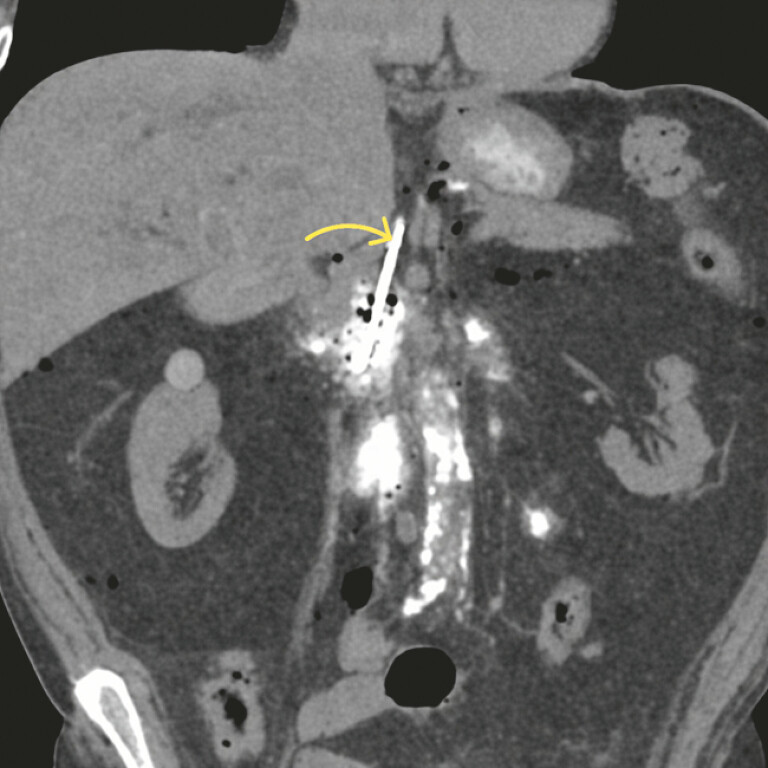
A CT image of the abdomen showing the malpositioned plastic biliary stent extending into the retroperitoneal space posterior to the pancreatic head with partial projection into the small bowel. CT, computed tomography.
